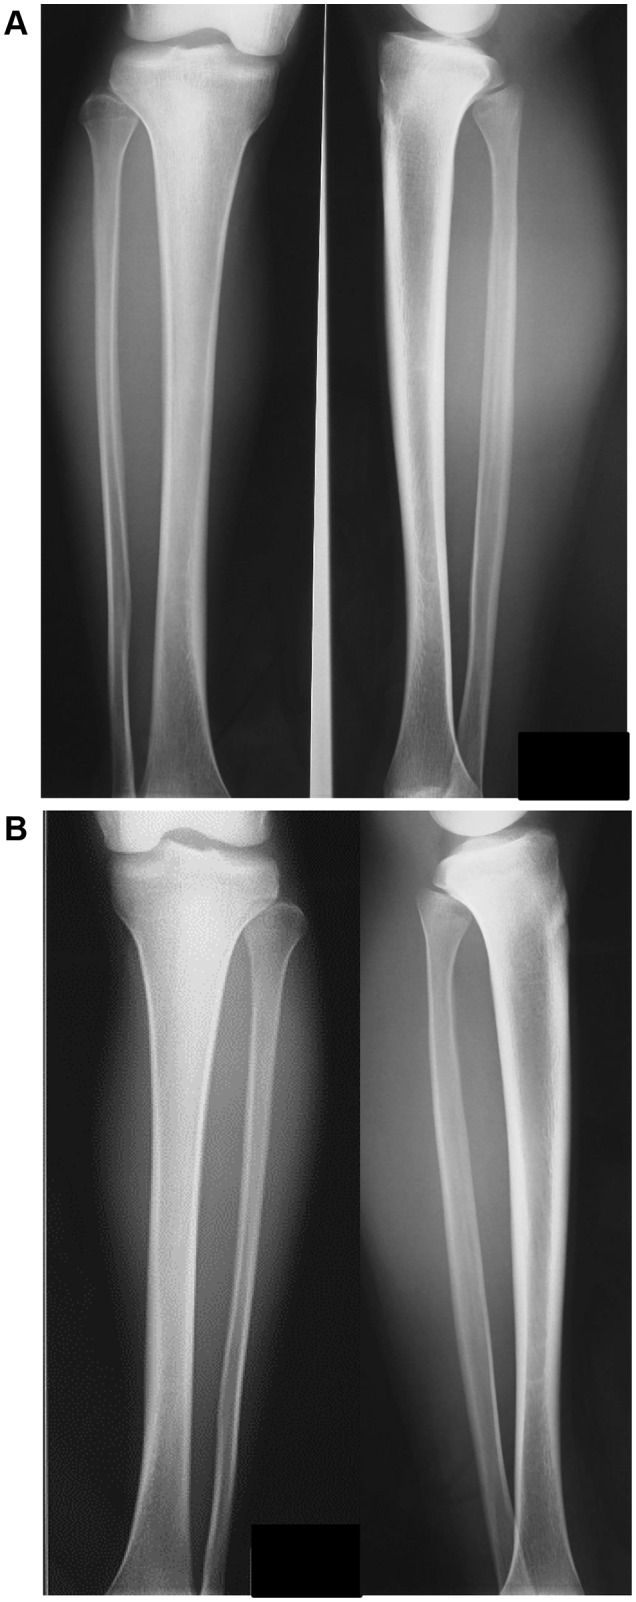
Initial radiographs show suspected tibial stress fracture or shin splints. There is no clear abnormality in the radiographs of the tibia in the antero-posterior and lateral views. A Right side; B left side
